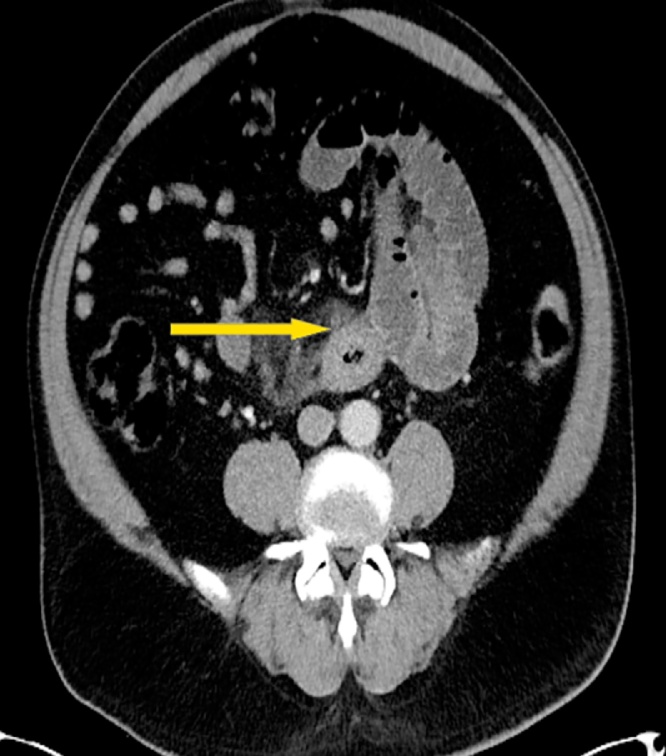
CT abdomen axial another view showing dilated small bowels along with mesenteric swirling; target sign (yellow arrow).
